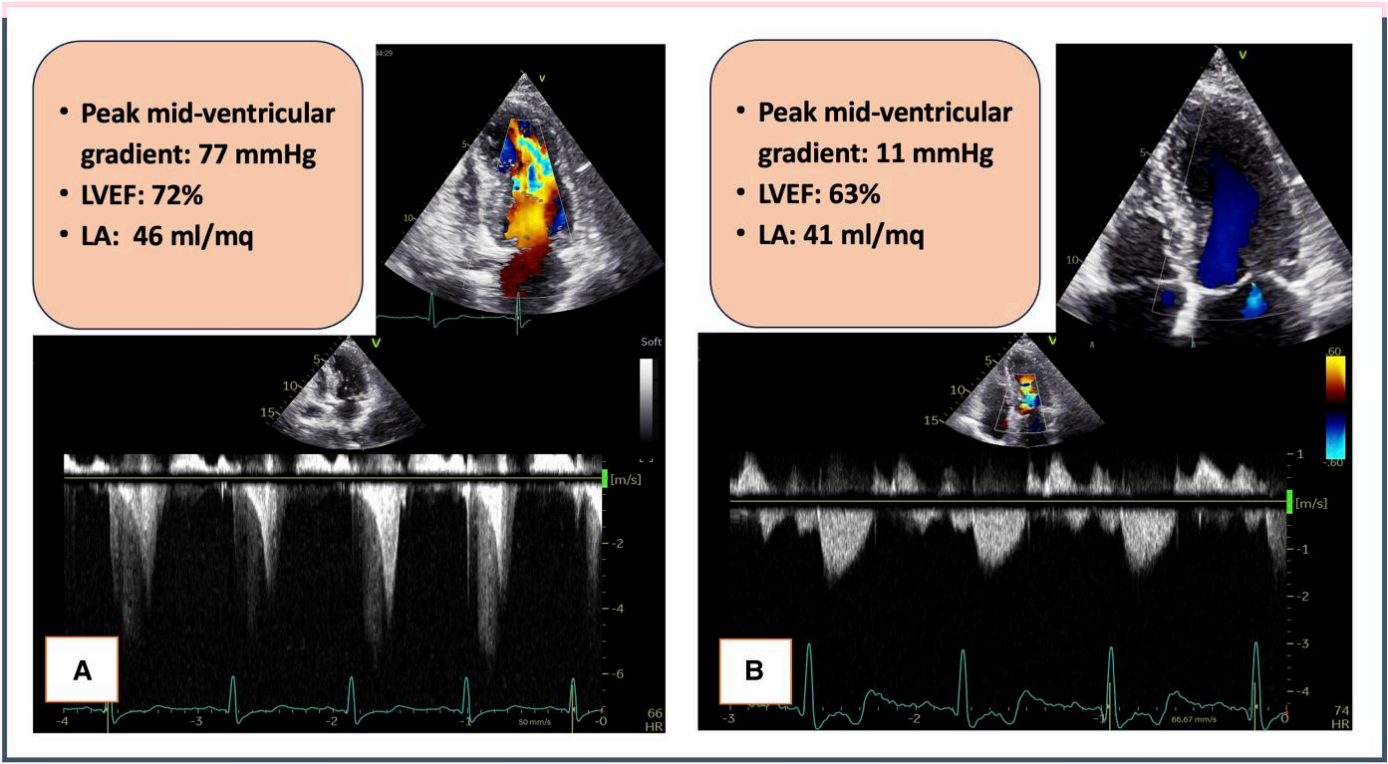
Echocardiographic characteristics at baseline and at 16 weeks follow-up in Patient 1. On the left side, baseline echocardiographic data are shown (*A*) while on the right side, data after 16 weeks of treatment with mavacamten are indicated (*B*). Note the differences in scale. LVEF, left ventricular ejection fraction; LA, left atrium.
